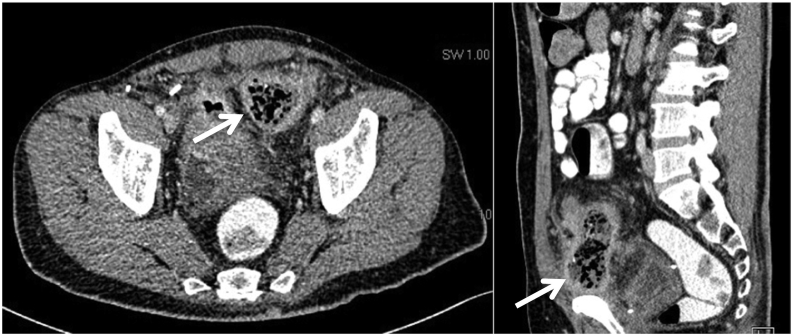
Abdominal computed tomography showed bowel wall thickening and inflammatory stranding involving the sigmoid colon (white arrows).
